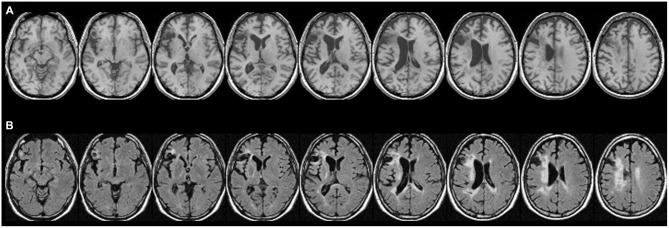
Patient CU’s anatomy in standard Montreal Neurological Institute (MNI) space on transverse slices. (A) Depicts a high-resolution T1-weighted structural MRI, while (B) shows a fluid-attenuated inversion recovery (FLAIR) scan. In most cases, T2-weighted images such as FLAIR scans show more extensive injury (e.g., gliosis) that is difficult to detect on T1-weighted scans. While large parts of Exner’s area and the ventral pathway are replaced by a pseudocyst following a colliquative necrosis, parts of the superior longitudinal fascicle (SLF II), might be affected by some gliosis (white tissue).
